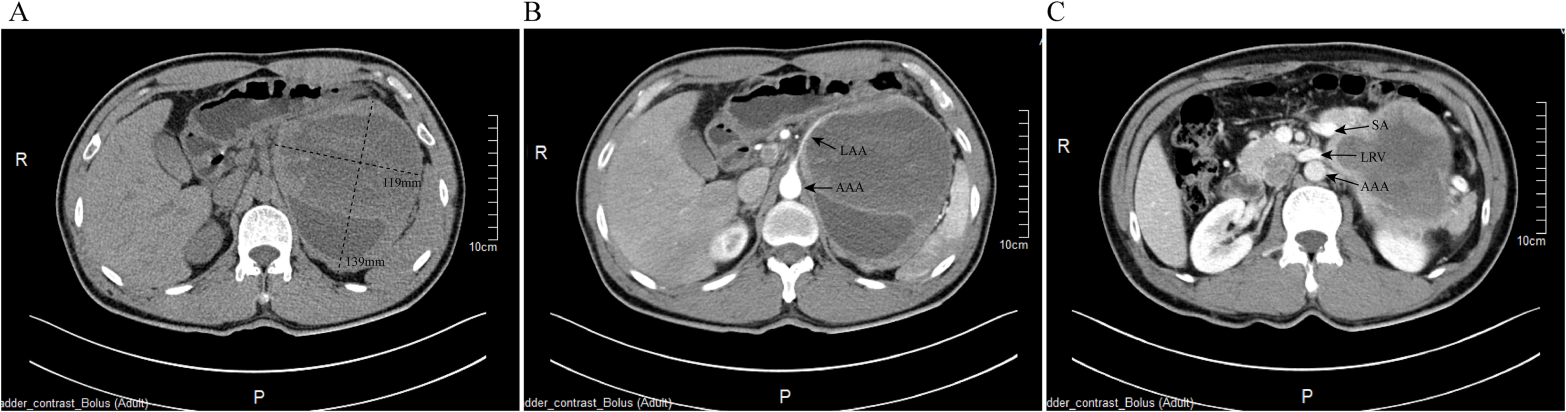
Preoperative contrast-enhanced CT imaging of the left adrenal pheochromocytoma. (A) Non-contrast axial CT scan showing the adrenal mass measuring approximately 119 mm × 130 mm; (B) Arterial phase imaging demonstrating the tumor’s vascular supply, with the left adrenal artery (LAA) originating from the abdominal aorta (AAA); (C) Venous phase imaging highlighting tumor involvement with surrounding vasculature, including the splenic artery (SA), left renal vein (LRV), and AAA.
